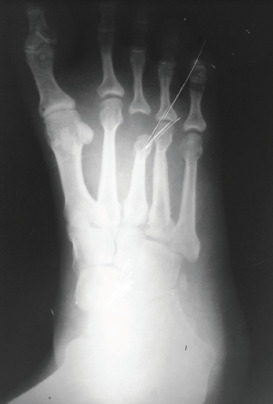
Radiograph of foot showing dislocation of 3rd MTP joint.
